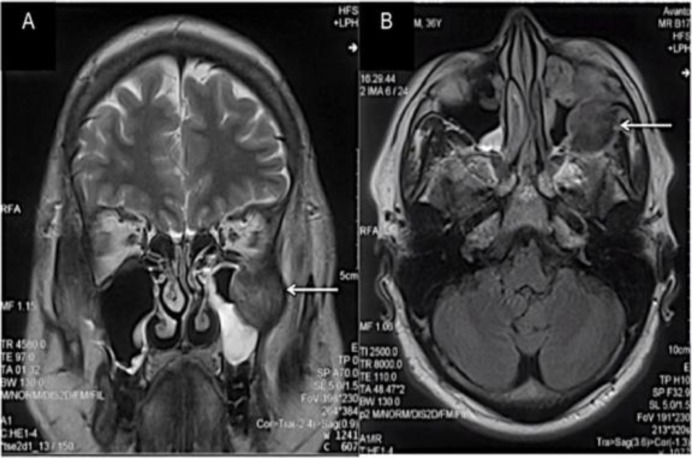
Coronal (A) and axial (B) MRI scans demonstrating a soft tissue lesion infiltrating the temporalis and lateral pterygoid muscle in the left infratemporal fossa
